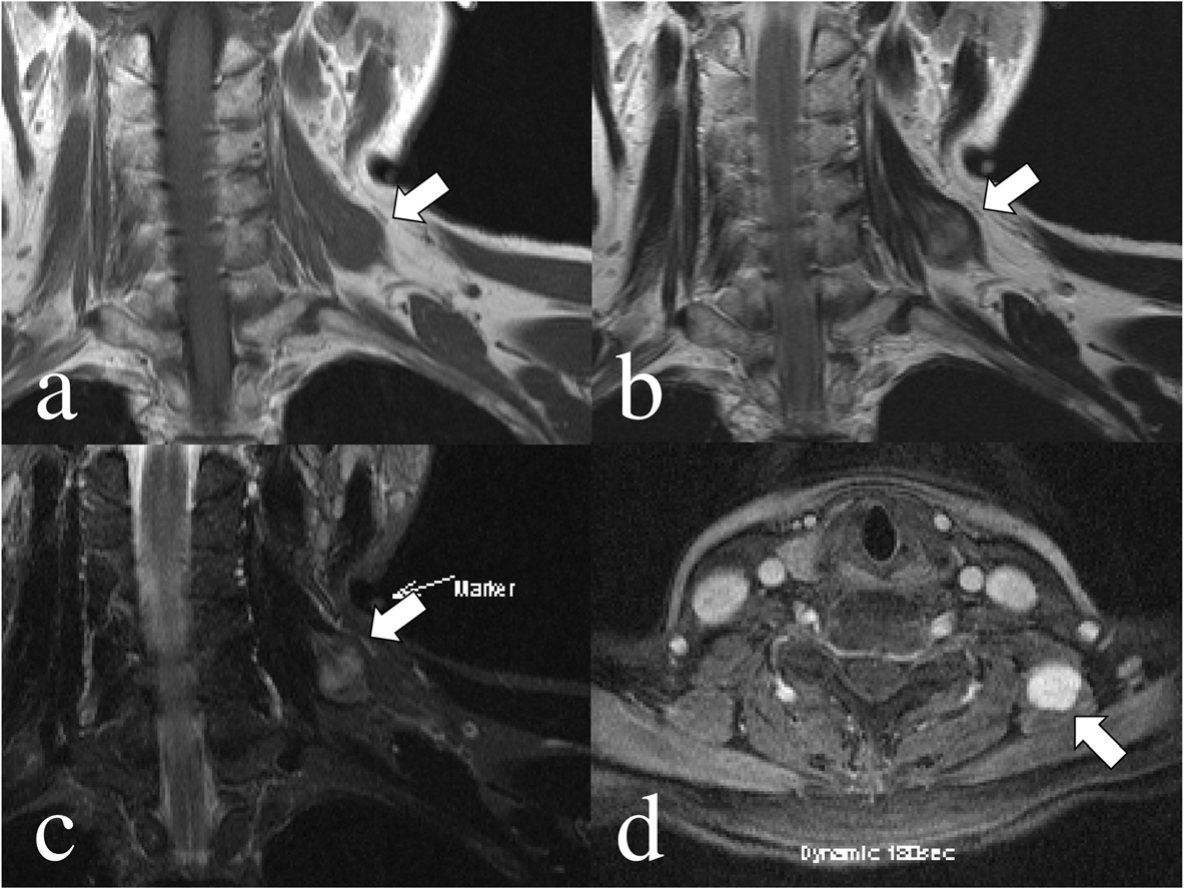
Preoperative magnetic resonance images (MRI) of the tumor. MRI revealed a tumor in the levator scapulae muscle. The mass with a size of 2.5 × 3.5 cm was well-demarcated and myxomatous (arrow). a T1 weighted image (WI). b T2WI. c short TI inversion recovery (STIR). d Contrast-MRI revealed good enhancement with gadolinium
